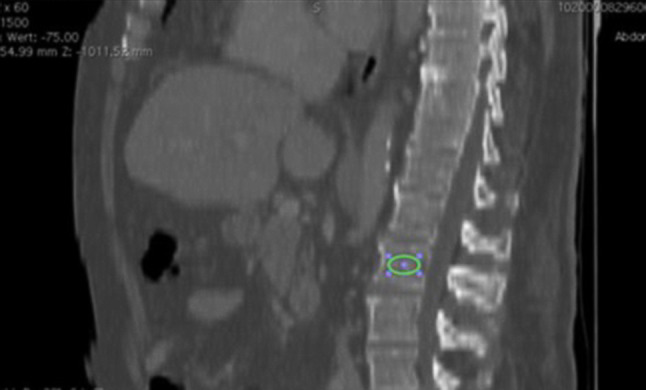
Sagittal cross-sectional X-ray image showing measurement of BMD at L1 (marked *green*)
